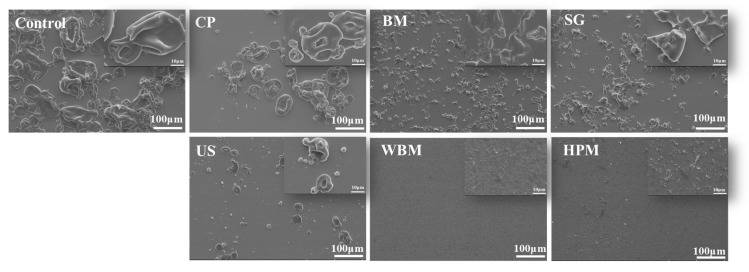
SEM images of walnut protein dry and wet routes of physical modifications (Control—control subjects; CP—cold plasma; BM—ball milling; SG—superfine grinding; US—ultrasound; WBM—wet ball milling; HPM—high-pressure microjet). (Magnification: large image—0.2 k; small image—2 k).
